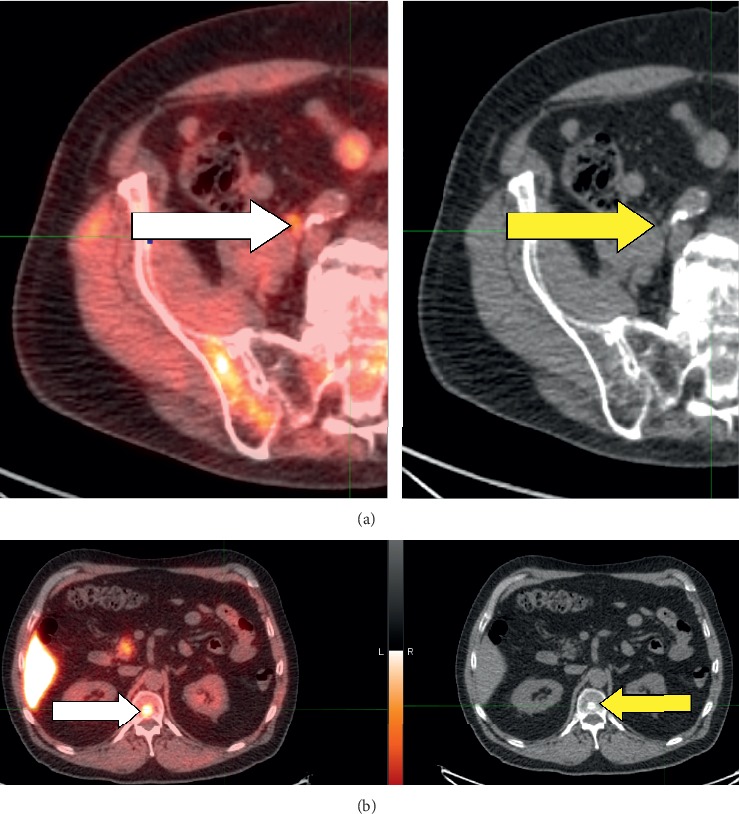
Images of an 80-year-old male who had received robotic-assisted laparoscopic prostatectomy and salvage radiation therapy. He was referred for ^18^F-fluciclovine imaging with PSA rising to 1.1 ng/mL. Top row: The axial, fused PET/CT image on the left identifies a focus of mildly enhanced ^18^F-fluciclovine uptake (white arrow) at the level of the common iliac vessels. The corresponding CT image on the right identifies 2 small, 5-mm lymph nodes (yellow arrow). Bottom row: note the ^18^F-fluciclovine-avid lesion in the body of T12 (white arrow, left-hand image), with the corresponding CT view on the right demonstrating the lesion at the identical location (yellow arrow).
